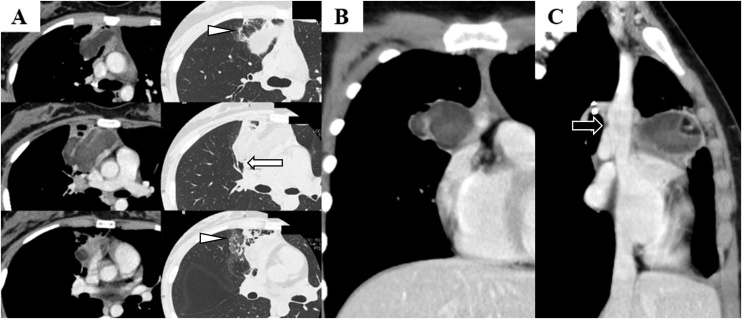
Enhanced computed tomography shows a 6.5 × 3.5-cm tumour with areas of fat density and a thick enhancing nodular wall on the mediastinal aspect. The B^3^ bronchus is obstructed by the tumour (white arrow) and there are ground glass opacities at the right upper lobe around the tumour (white corn). (A. Horizontal view). The tumour is contiguous to the superior vena cava (black arrow), but there are no oppressed vessels. (B. Coronal view and C. sagittal view)
